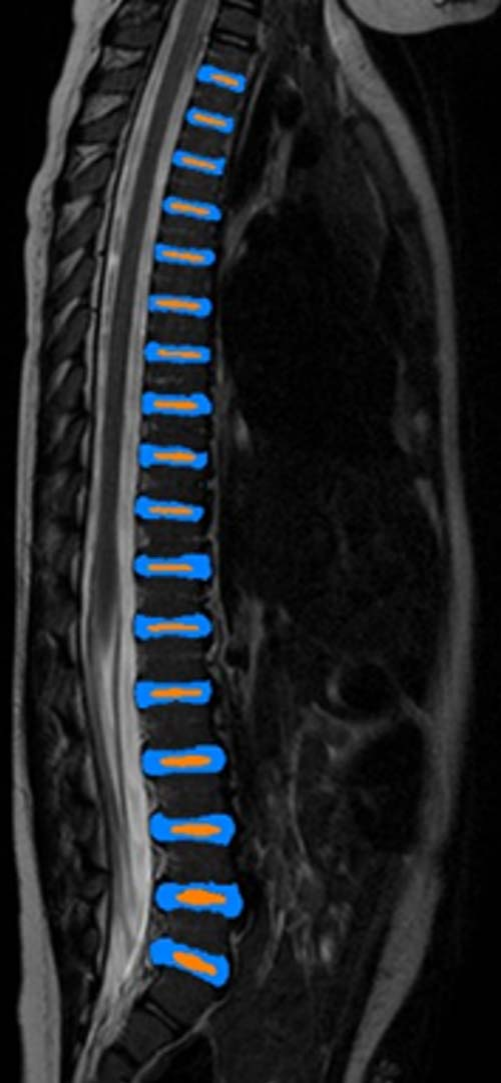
T2-weighted magnetic resonance imaging scan. Example of a segmentation of T1–L5. Nucleus pulposus in orange and annulus fibrosus in blue.
